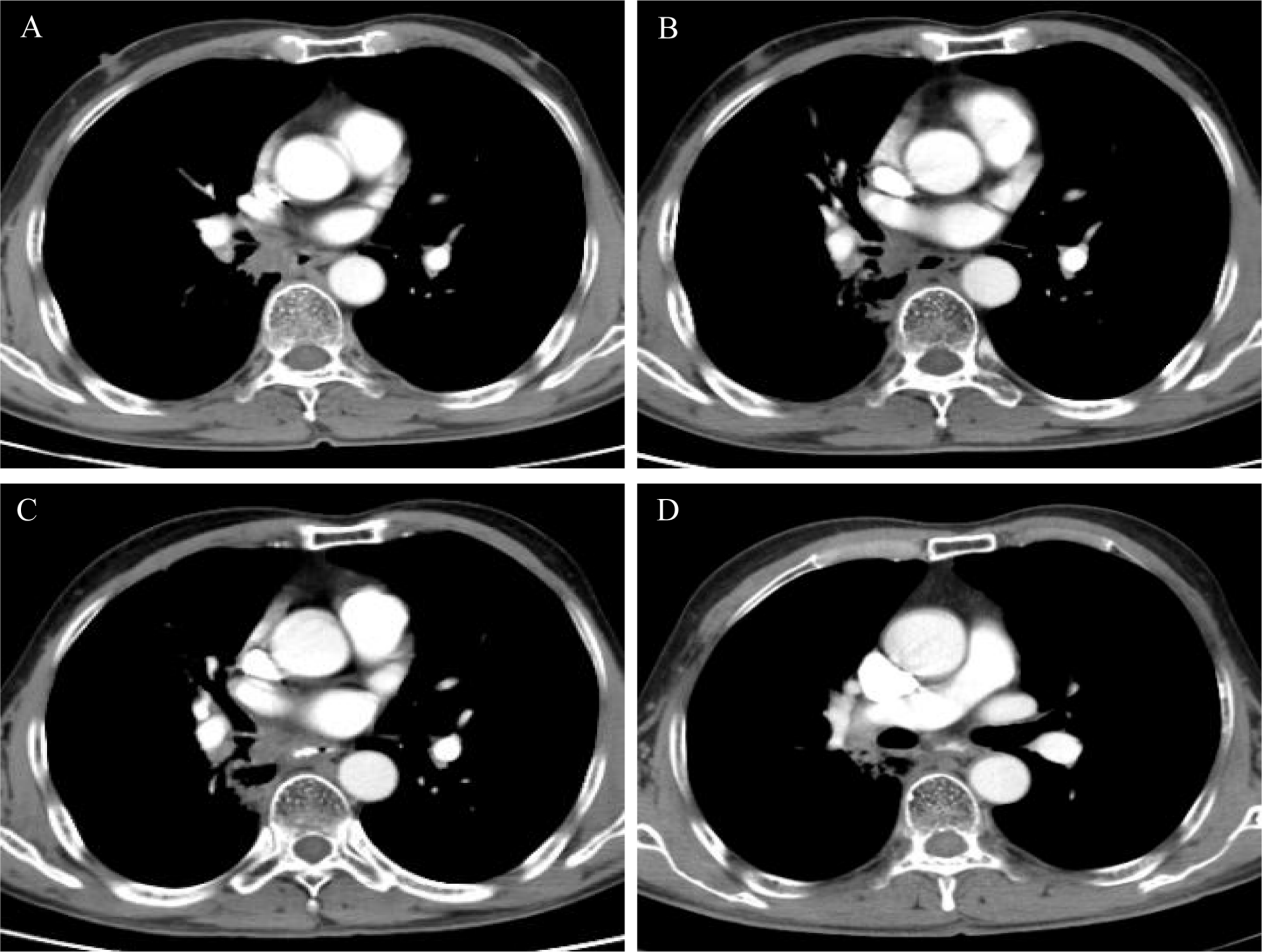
(A) Computed tomography (CT) scan after chemoradiation showed partial response; (B–D) CT scan showed stable disease during osimertinib treatment.
